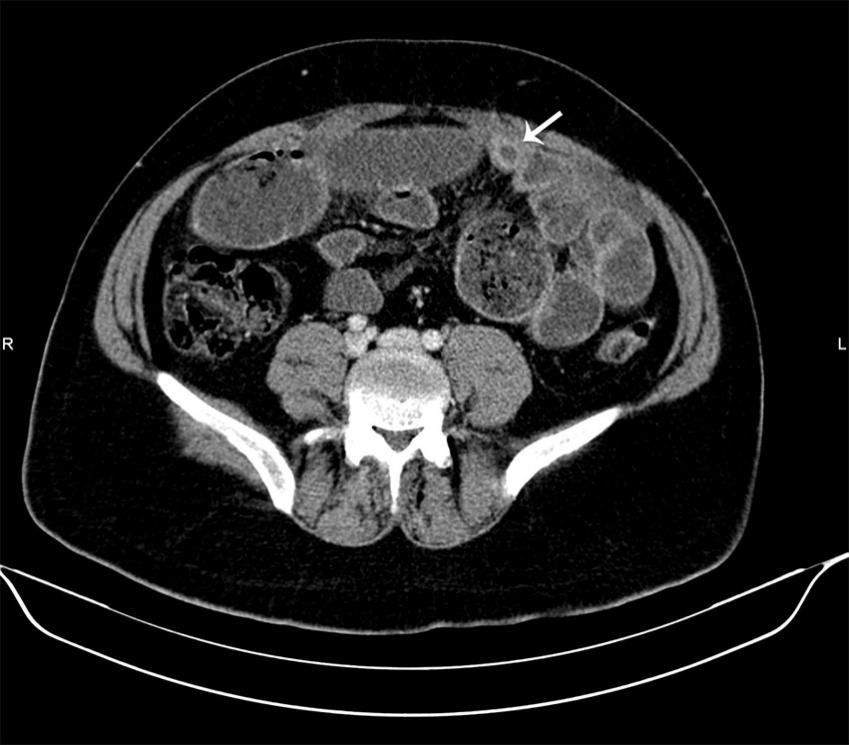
An axial CT image reveals a fluid-filled tubular structure in the lower abdomen (arrow).
